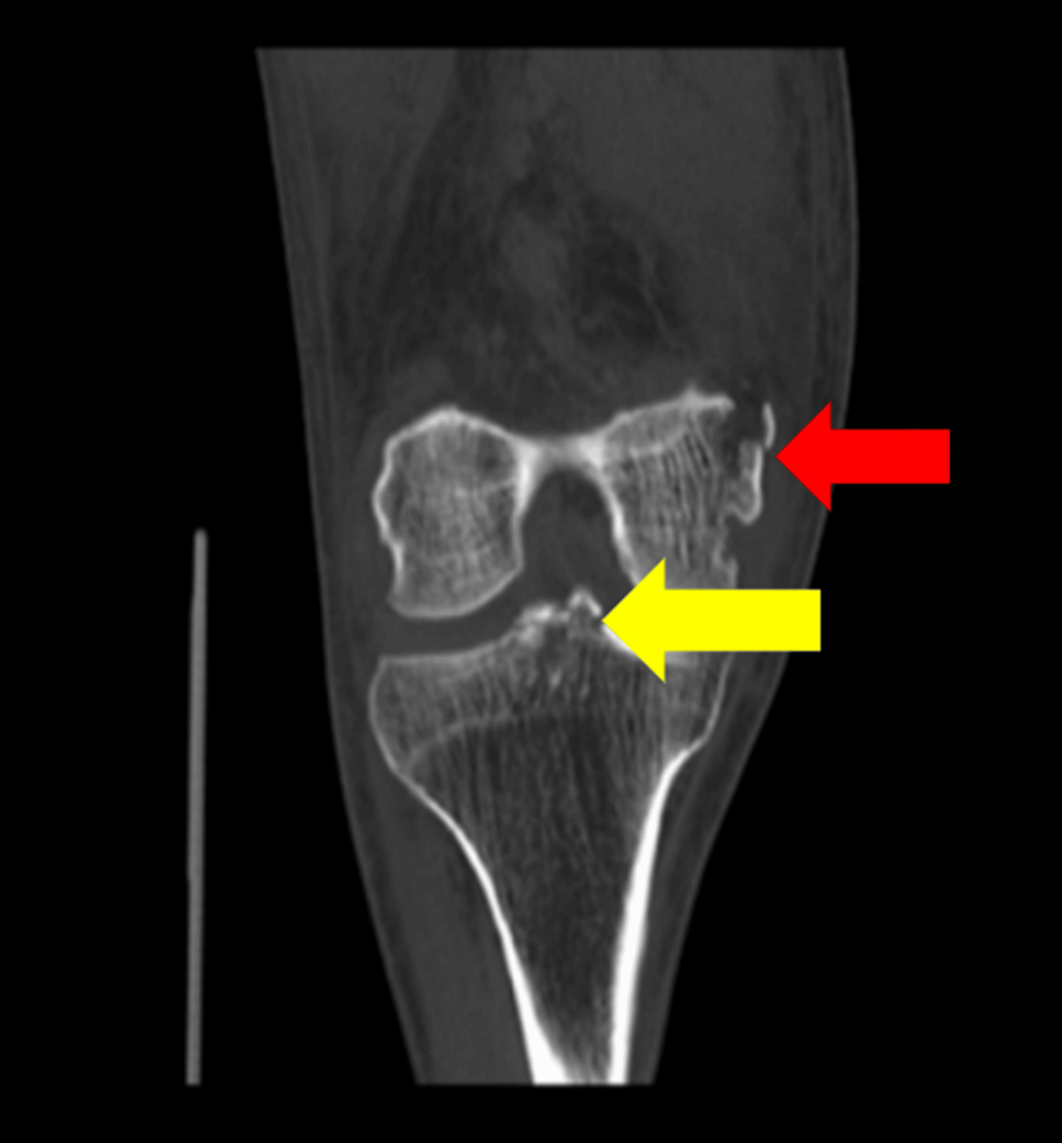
CT scan images of the right knee. The red arrow indicates a fracture of the medial femoral epicondyle, and the yellow arrow highlights an avulsion fracture of the tibial eminence consistent with anterior cruciate ligament (ACL) avulsion.
